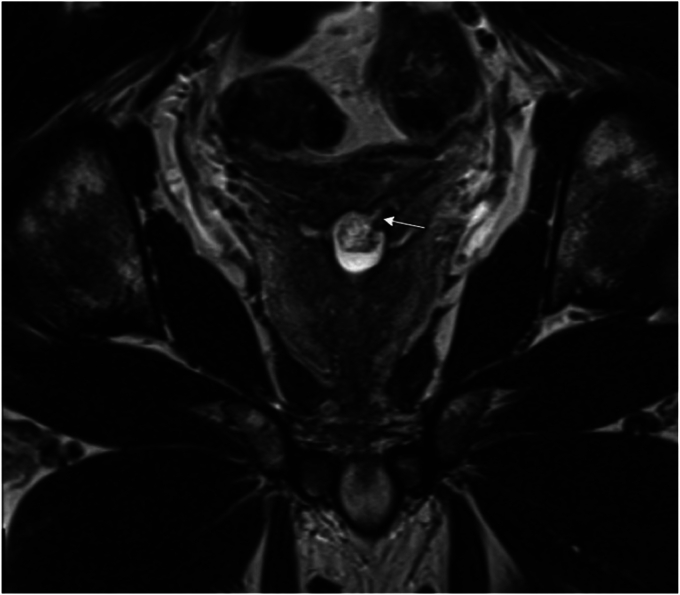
In a 35-year-old transgender woman presenting with hematospermia, magnetic resonance imaging revealed a 1.7-cm posterior prostatic cyst communicating with the left seminal vesicle.
